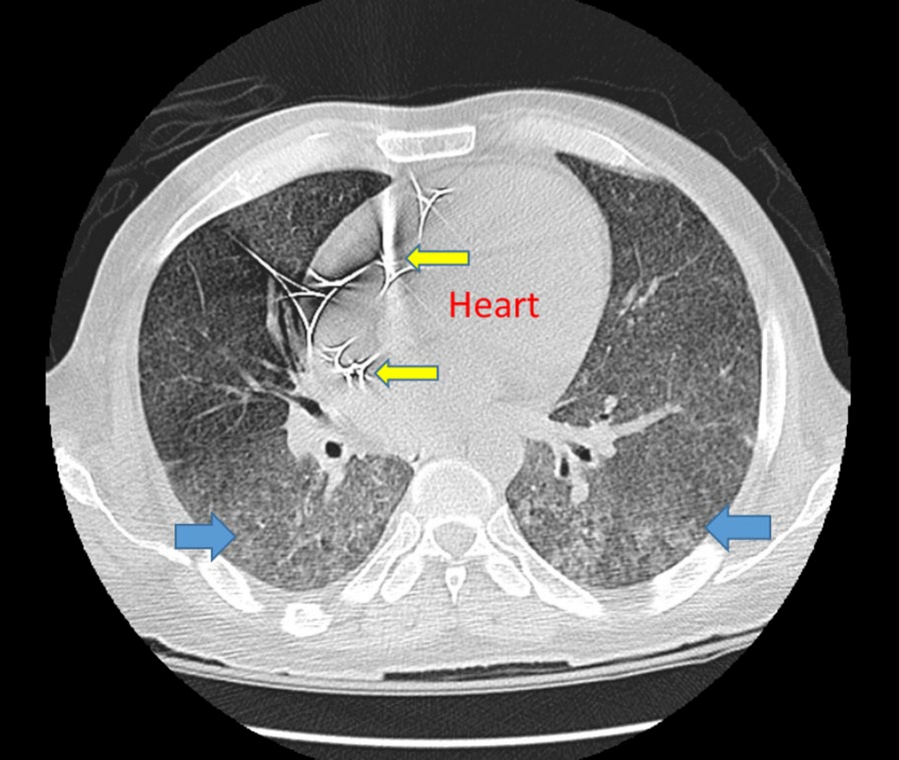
Computed tomography (CT) chest axial plane showing diffuse randomly distributed ground-glass nodules (*blue arrows*) involving bilateral lungs consistent with military tuberculosis. Implantable cardioverter defibrillator leads seen (*yellow arrows*)
